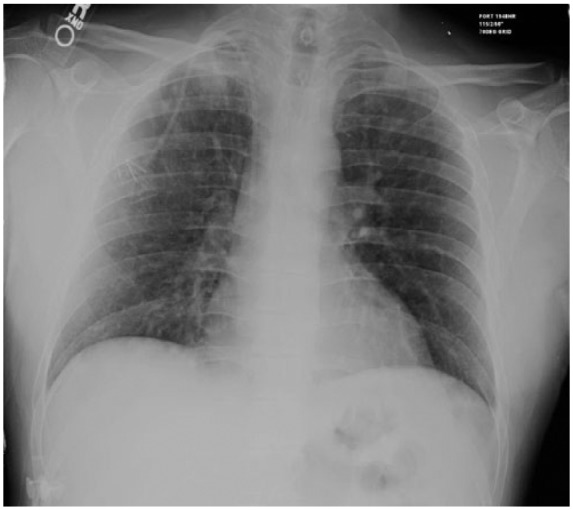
Admission imaging. Chest X-ray with evidence of bilateral airspace opacities.
